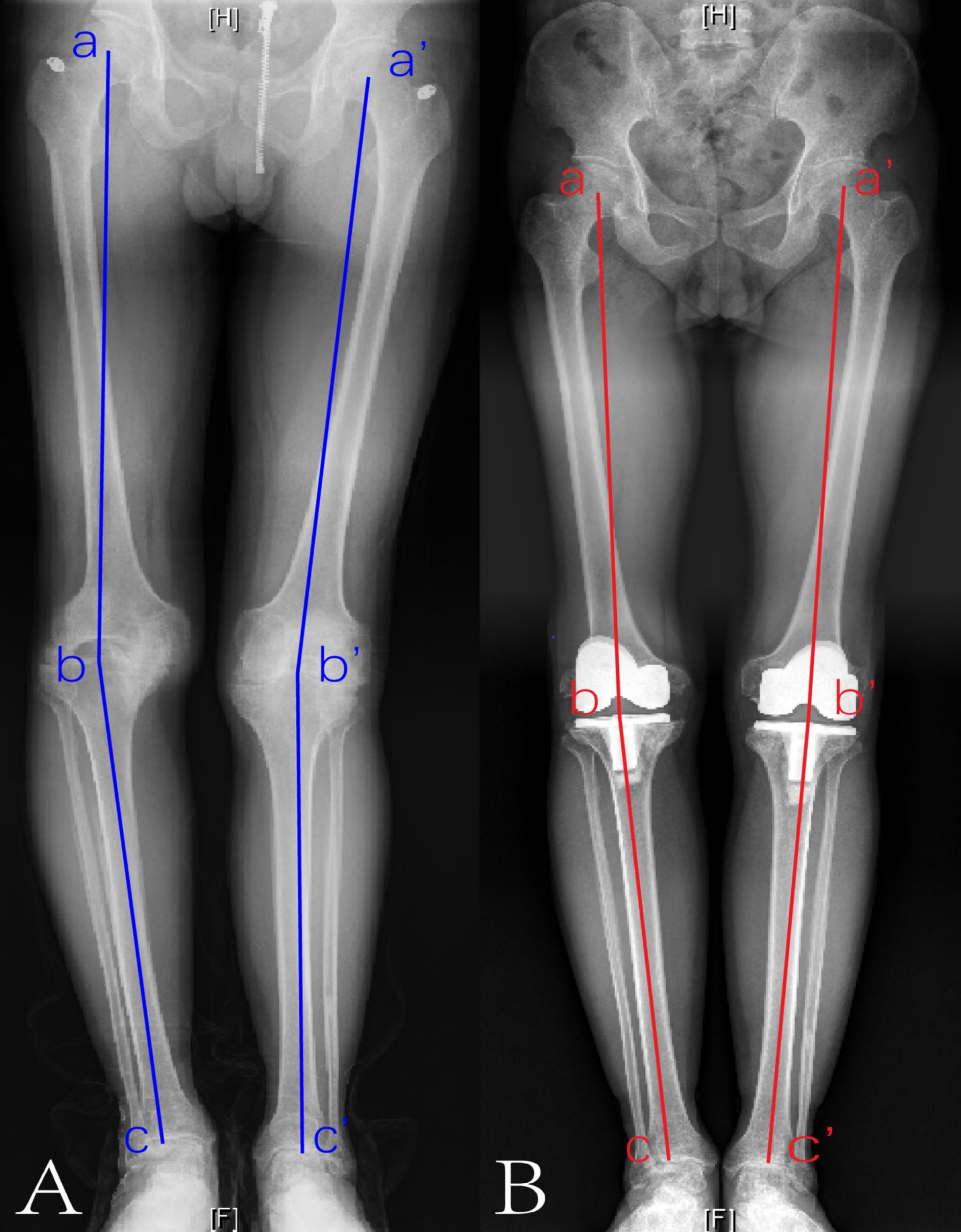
Long-leg standing radiographs of a male patient with advanced bilateral haemophilic knee arthropathy. (A) Preoperative anteroposterior view. Blue line: the preoperative mechanical axis. (a and a’: the centre of the right and left femoral head, respectively; b and b’: the centre of the right and left knee, respectively; c and c’: the centre of the right and left ankle, respectively.) (B) The latest follow up anteroposterior radiograph after total knee arthroplasty twice. Red line: the postoperative mechanical axis. (a and a’: the centre of the right and left femoral head, respectively; b and b’: the centre of the right and left knee, respectively; c and c’: the centre of the right and left ankle, respectively.)
